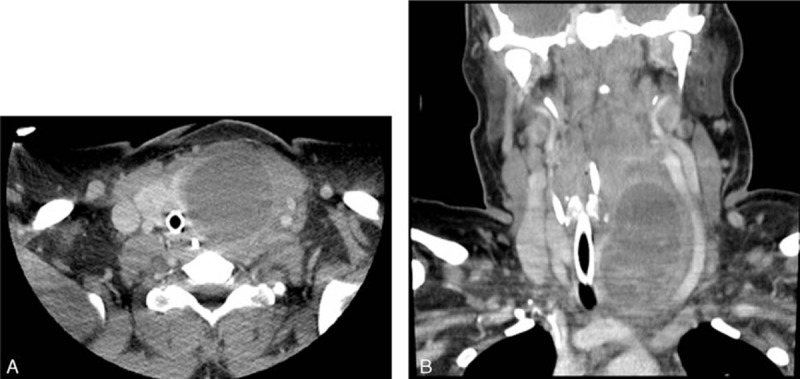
(A) Contrast-enhanced axial CT scan demonstrating left intrathyroidal hematoma. (B) Contrast-enhanced coronal CT scan demonstrating tracheal deviation and compression from left intrathyroidal hematoma.
